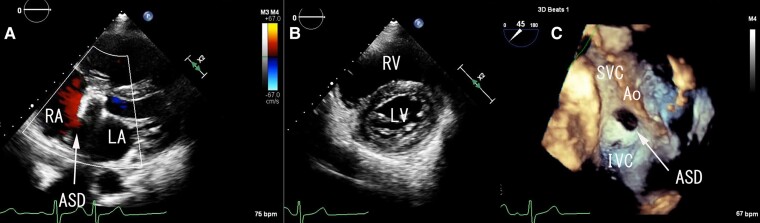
Atrial septal defect diagnosis in echocardiogram. (*A*) Secundum atrial septal defect and left-to-right shunt in transthoracic echocardiogram. (*B*) Short-axis view in transthoracic echocardiogram during the diastolic phase. It is considered that there was right ventricular volume overload due to the left ventricle being compressed during the diastolic phase. (*C*) Crescent-shaped secundum atrial septal defect with aortic and superior rim deficiency in transoesophageal echocardiogram. Videos of (*A*)–(*C*) are also provided in Supplementary material online, *Video S1A–C*. RA, right atrium; LA, left atrium; ASD, atrium septal defect; RV, right ventricle; LV, left ventricle; Ao, aorta.
